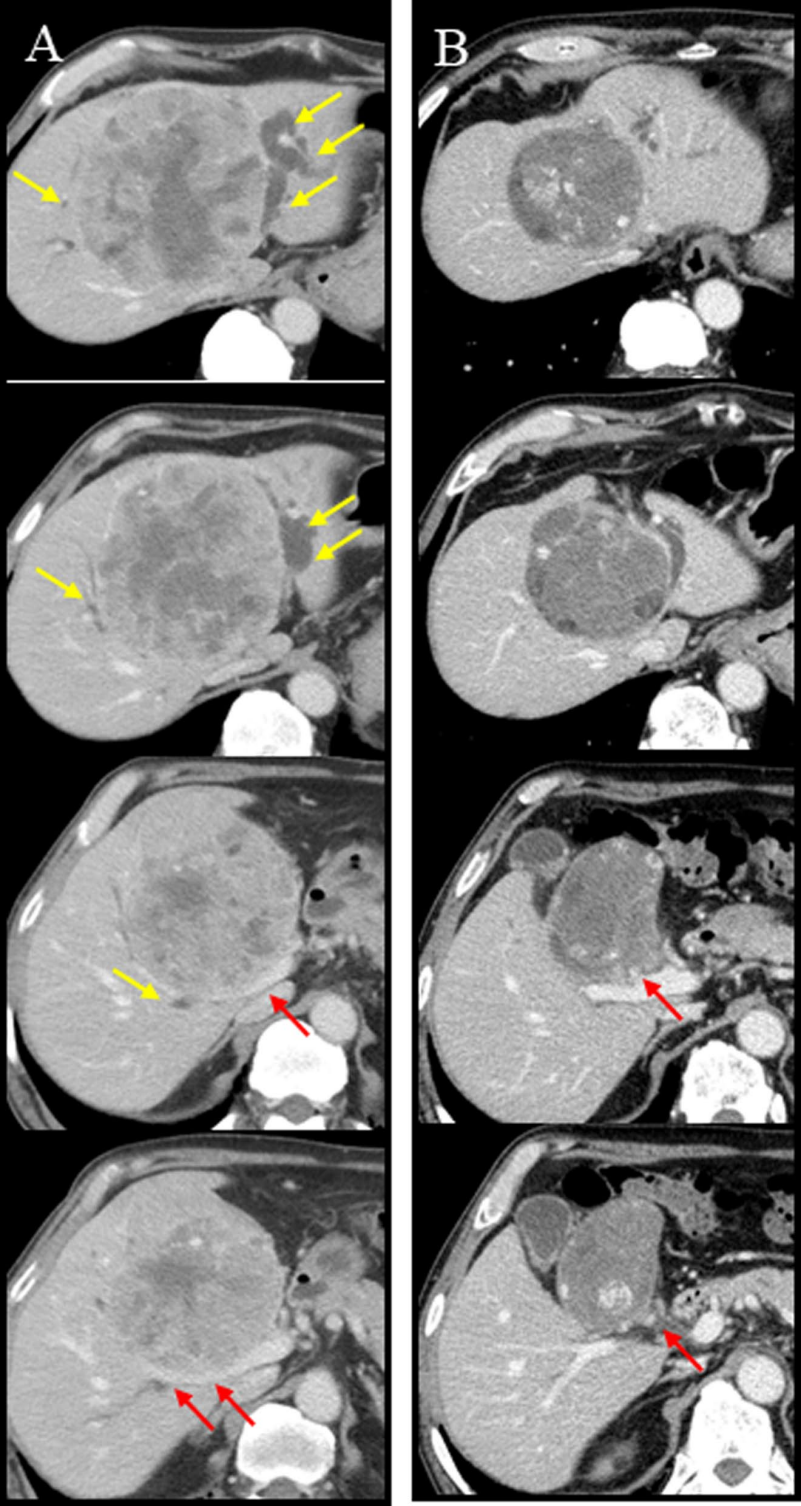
Computed tomography of case 1. A Neoplastic lesion in S4 at the time of diagnosis. Yellow arrows show dilatation of intrahepatic bile duct in anterior, and lateral segment. Red arrows show tumor contact with right hilum region. B Neoplastic lesion in S4 after course of ATEZO/BEV. Tumor contact with hilum region regressed to only left hilum (red allows)
